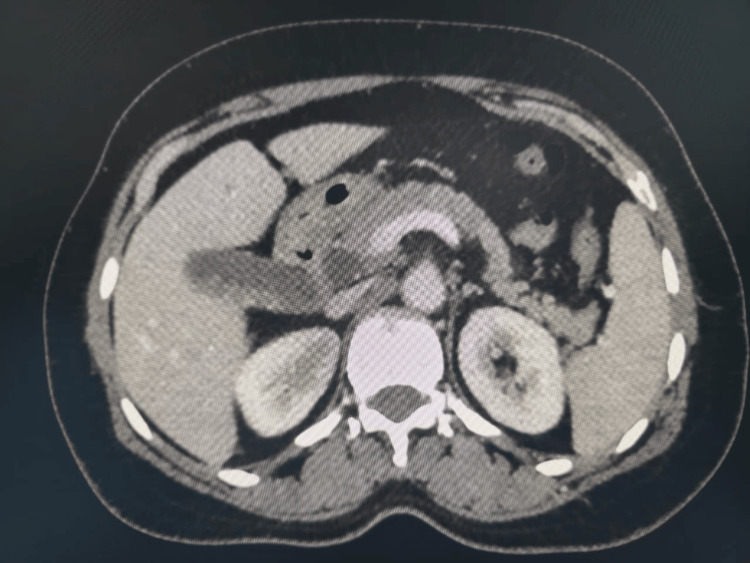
CT image showing dilatation of the extrahepatic biliary duct CT: computed tomography
